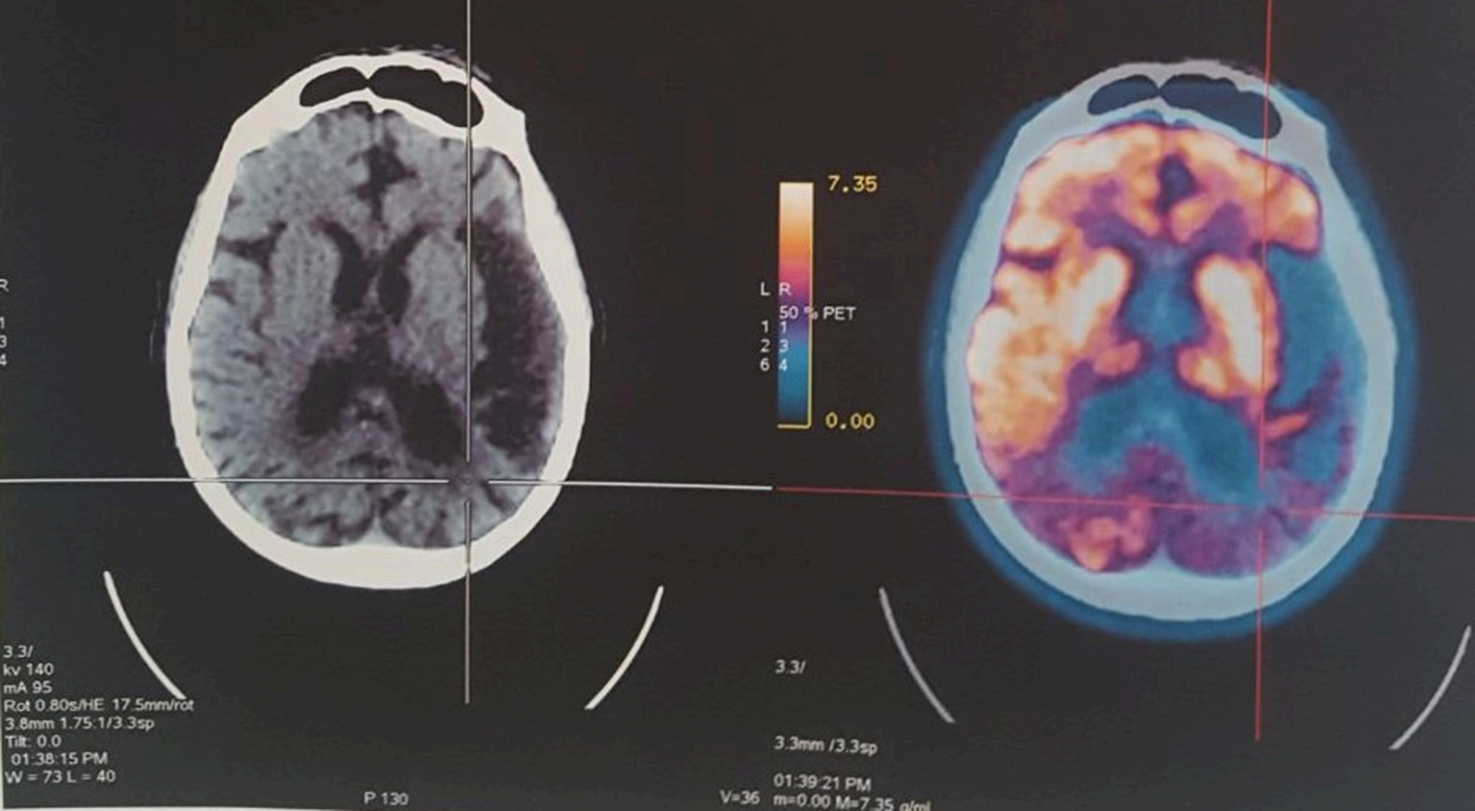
PET-CT revealed hypometabolism and atrophy in the left temporal lobe and posterior region of the parietal lobes.
